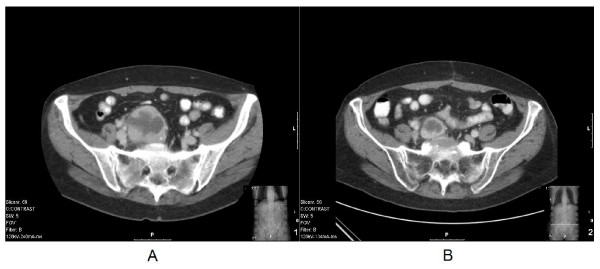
A: Computed Tomography (CT) of the abdomen showing the tumour and its relationship with the sacral vertebrum, the adipose tissue and the common iliac artery and vein, before neo-adjuvant radiotherapy. B: Computed Tomography (CT) of the abdomen showing the marked downsizing of the tumour, after neo-adjuvant radiotherapy.
